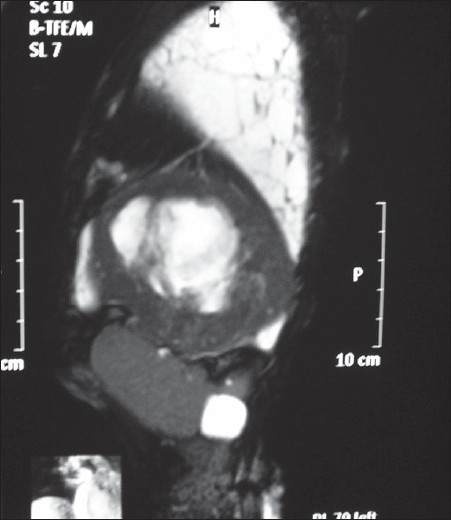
T2-weighted sagittal MRI image
